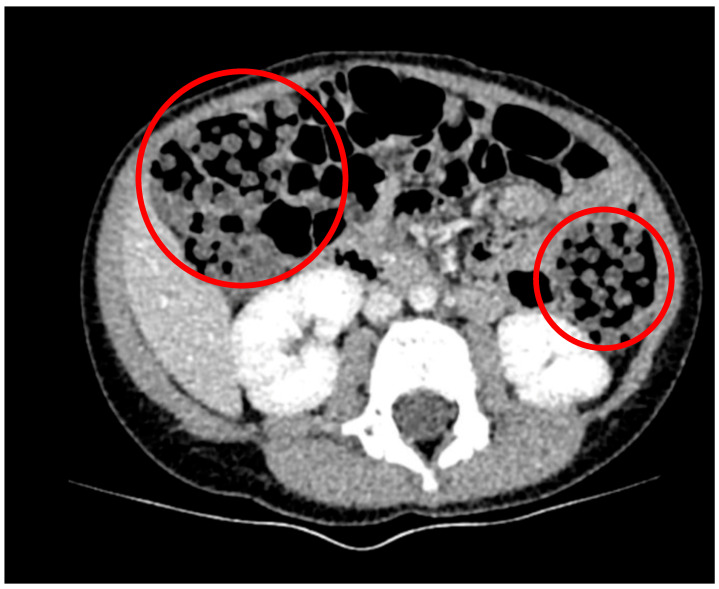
Computed tomography scan on a 36-month-old patient after water bead ingestion, managed nonoperatively. Circles indicate ingested beads.
